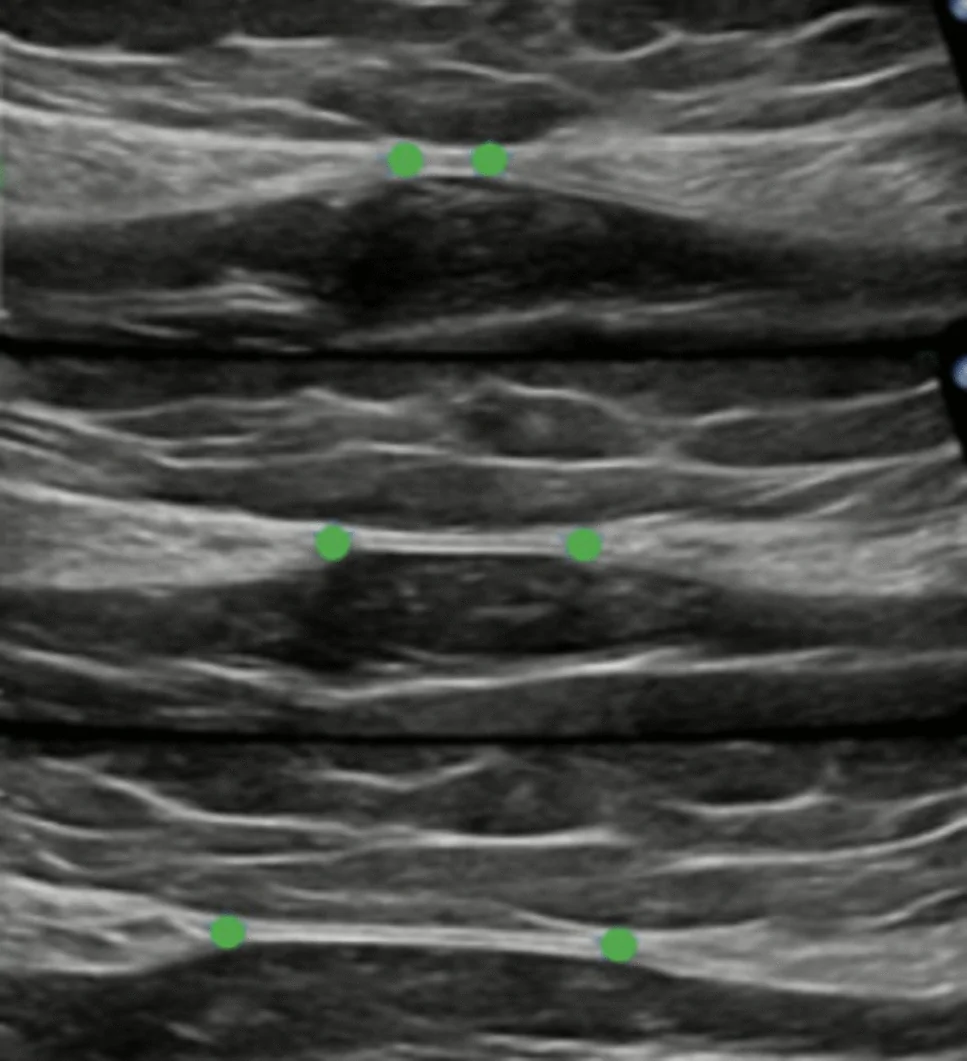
Intraoperative ultrasound image. The three standardized measurements used for evaluating the abdominal wall are highlighted in green. These include the width of the rectus diastasis at the supraumbilical, umbilical, and infraumbilical levels. Intraoperative ultrasound serves as a dynamic and precise tool for assessing the extent of the diastasis, marking it and guiding the surgical strategy, especially in cases involving minimally invasive or hybrid techniques
